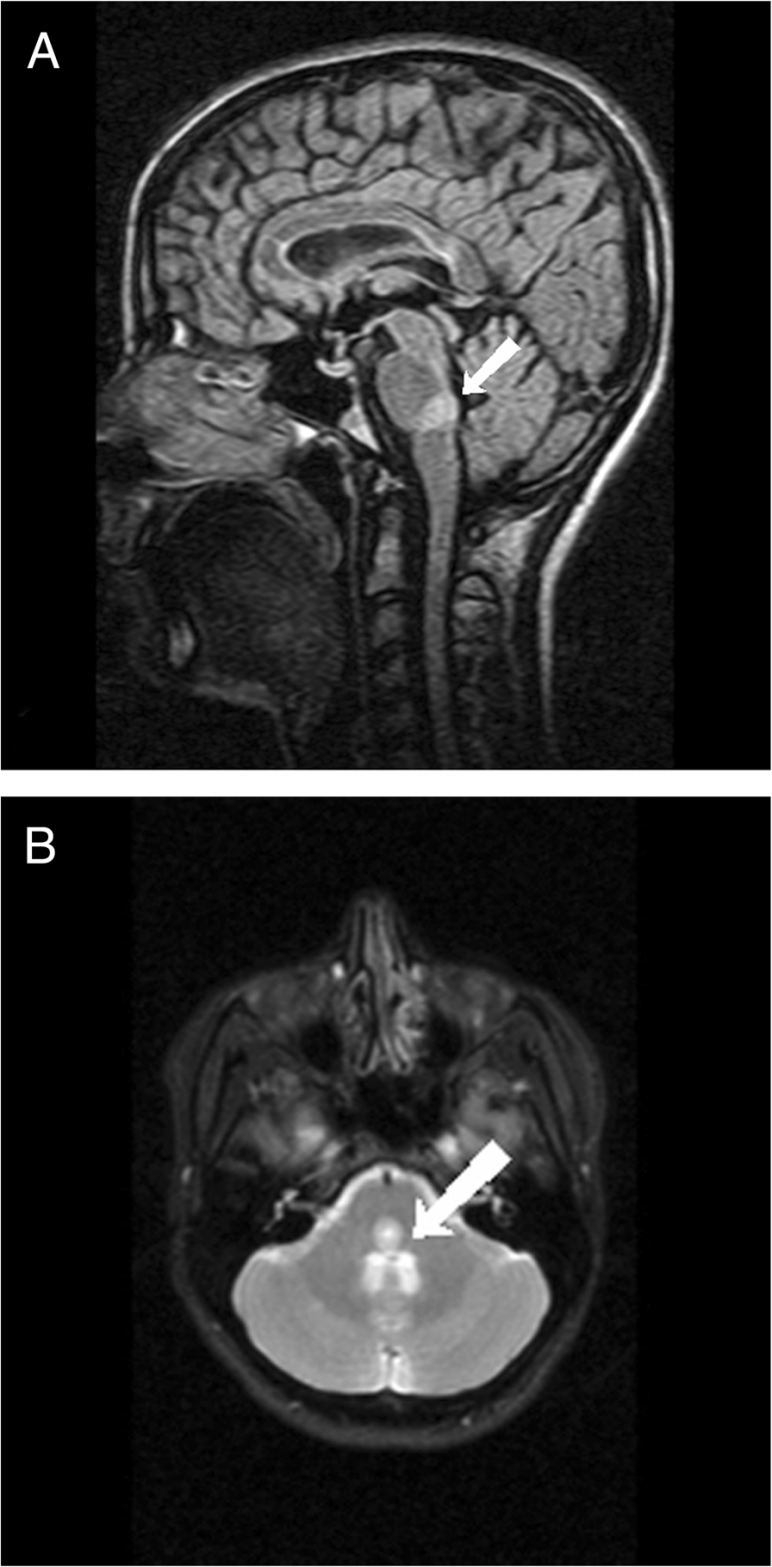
a Sagittal FLAIR MRI showing a hyperintense lesion (arrow) in the PPRF area. b Axial T2-weighted MRI showing bilateral hyperintensities (arrow) in the PPRF area in the pons at the approximate level of the abducens nucleus and facial colliculus
